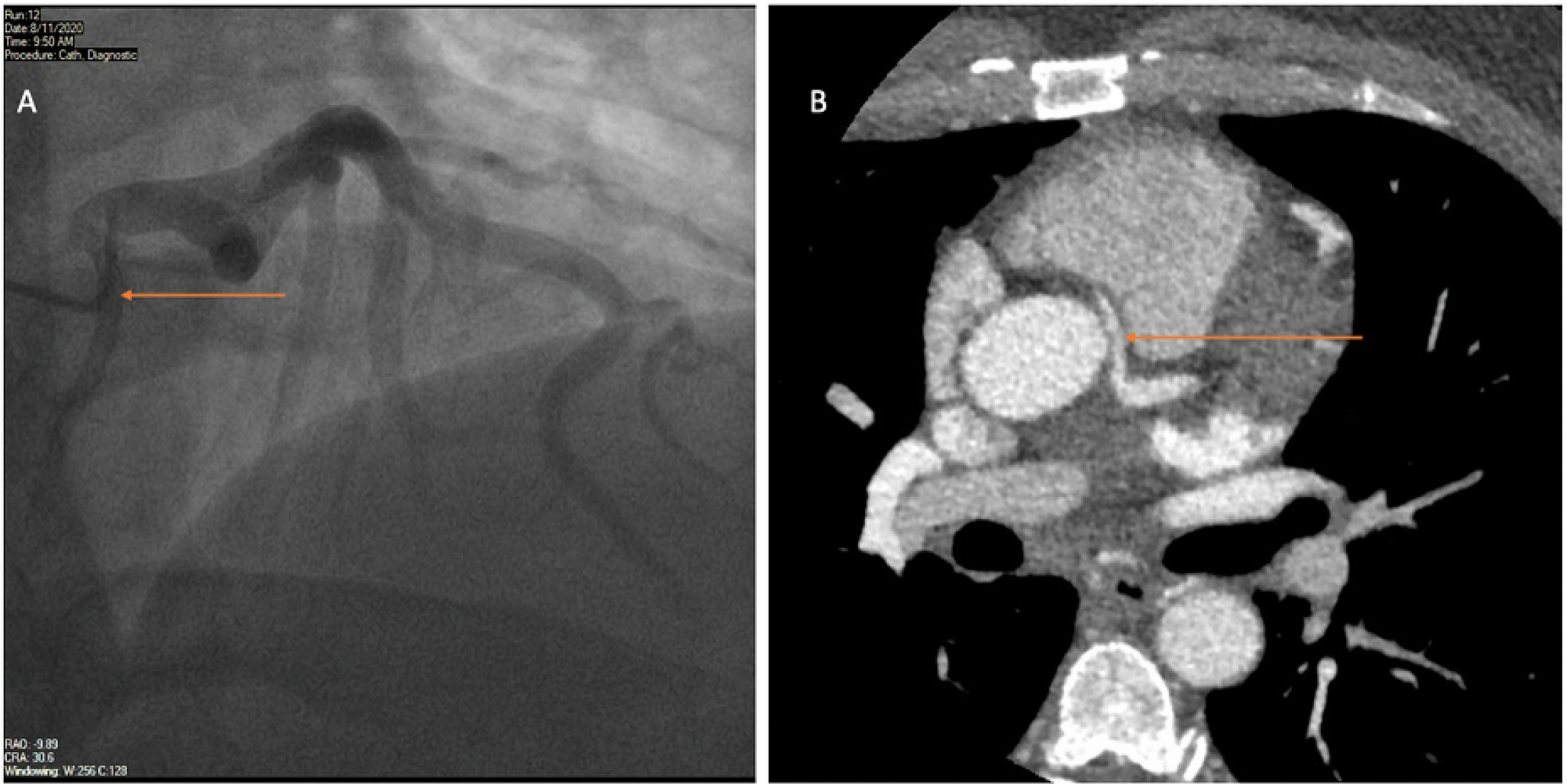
Coronary and CT angiograms A: Coronary angiogram with right anterior oblique (RAO) cranial view showing right coronary artery offsetting from the left main coronary artery ostium. B: Computed tomography (CT) angiogram showing the course of the anomalous right coronary artery between the aorta and pulmonary artery.
